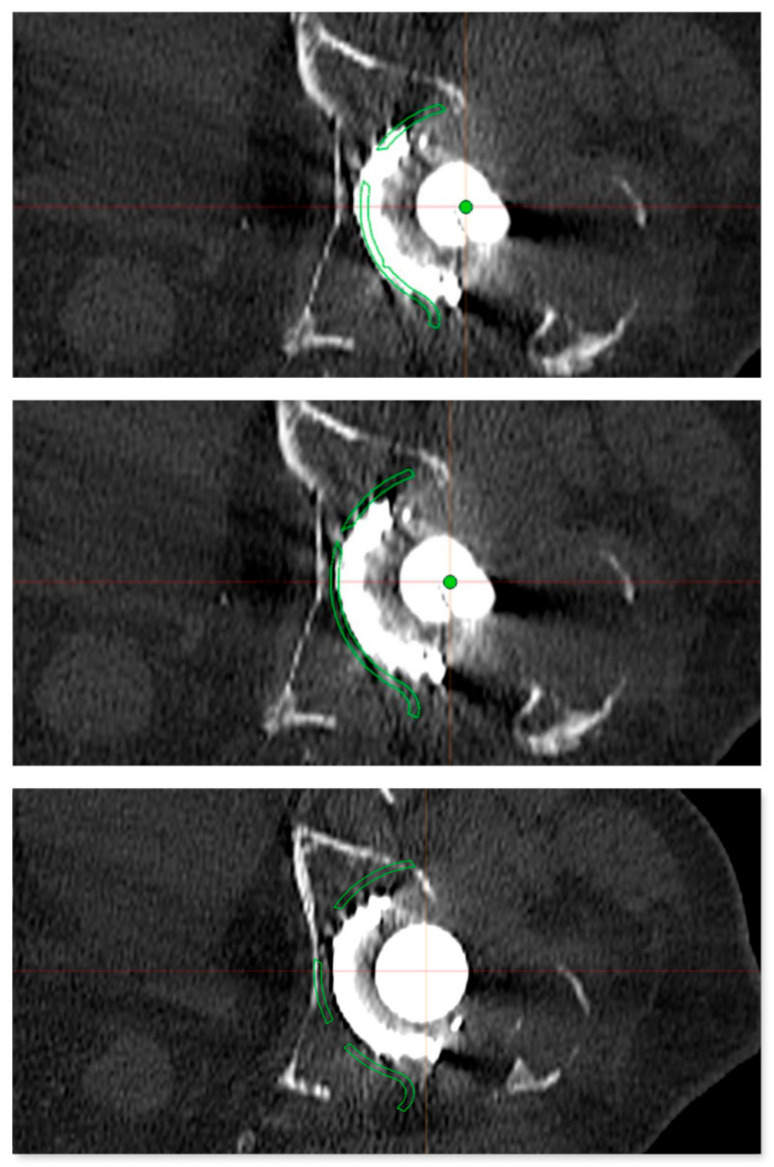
Preoperative planning of the reinforcement cage (Burch Schneider) in the transversal plane of the CT scan. The results of the personalized planning are shown in the center image, indicative of a Burch Schneider reinforcement cage 56 mm in diameter. The upper and lower images show reinforcement cages one size smaller (50 mm; top) and one size larger (62 mm, bottom). In this patient, a 56 mm size reinforcement cage was implanted.
